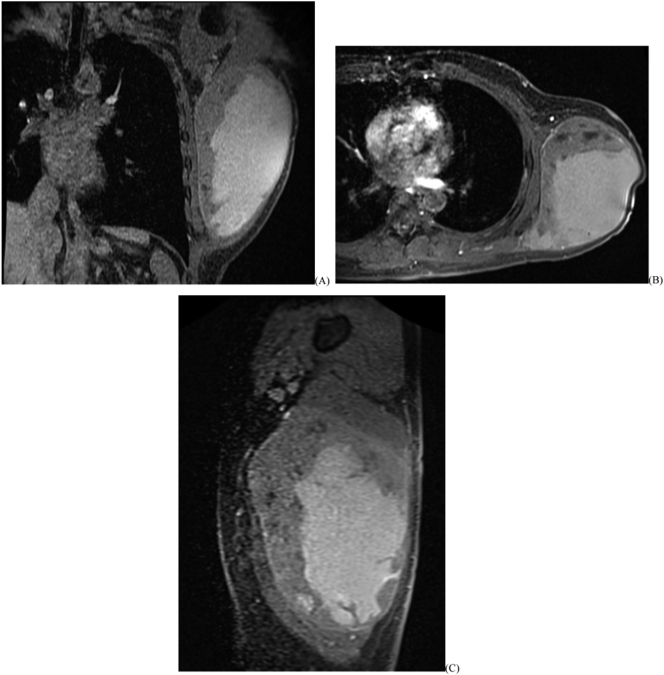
Patient 2 T1 fat-saturated MRI; (A) coronal; (B) axial; (C) sagittal.
